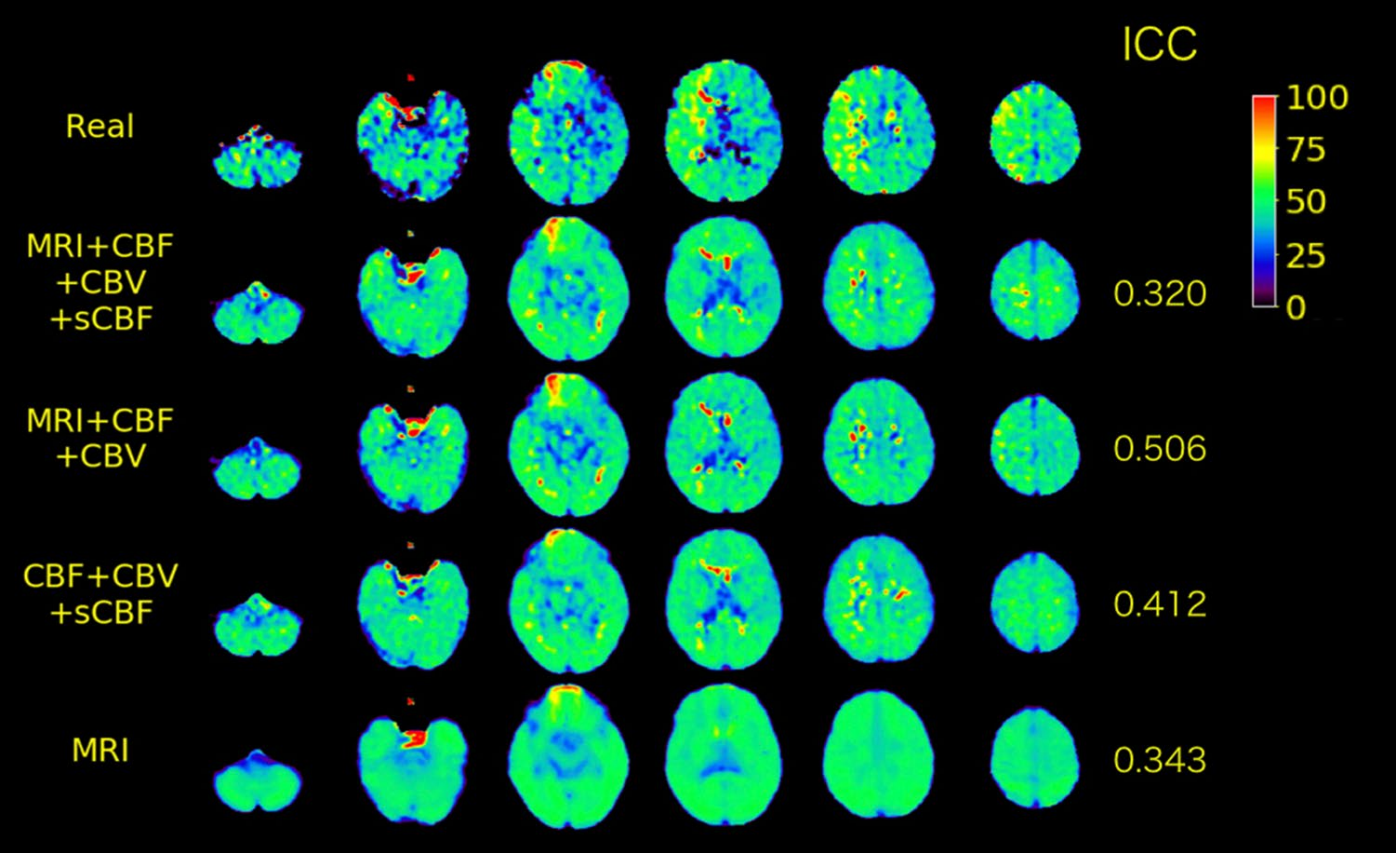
Real and predicted OEF maps for a case (71 years old, male, right internal carotid artery stenosis) with the lowest ICC by the full model (MRI + CBF + CBV + sCBF) among the validation data set. The legends are the same as those in Fig. 3
